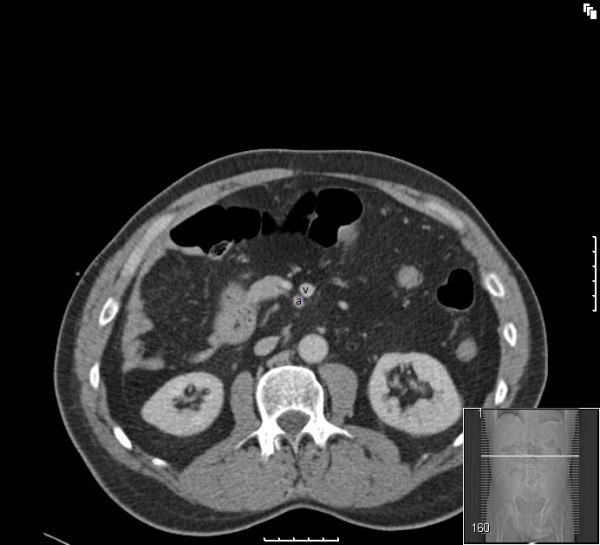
CT scan showing inverse relationship of SMA to SMV (a-artery and v-vein).
